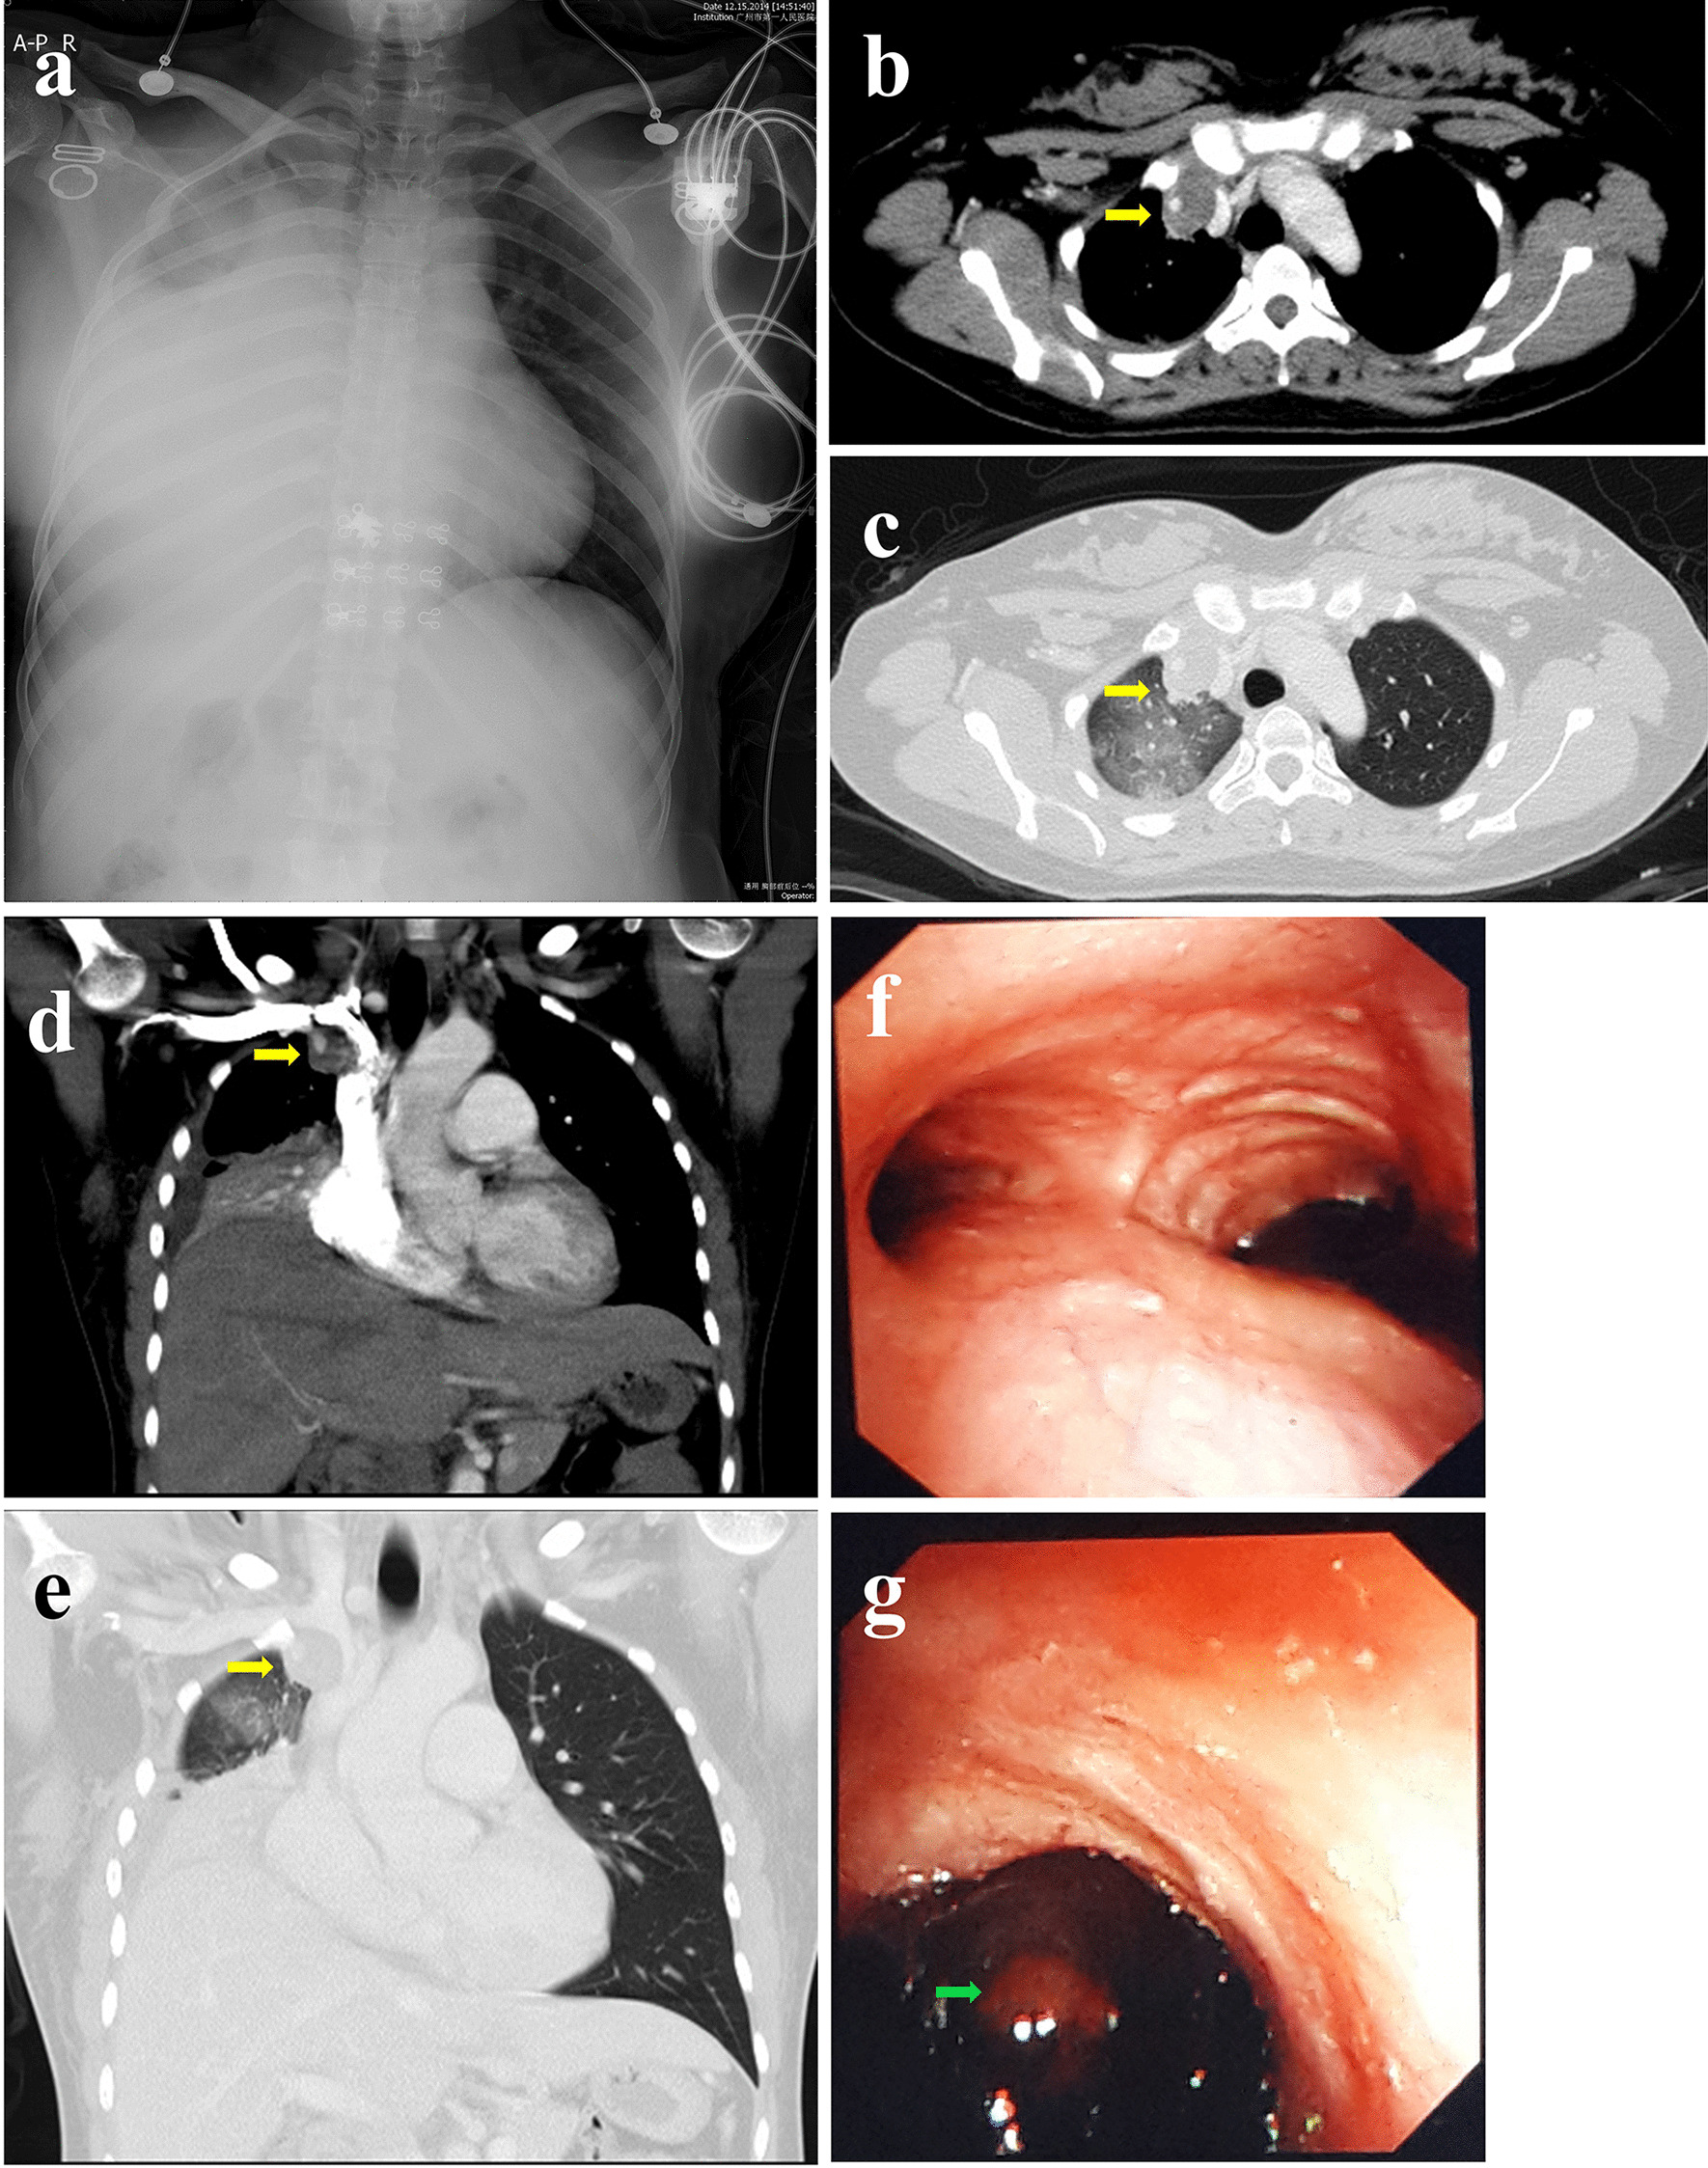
Chest radiograph indicating atelectasis of the right middle and lower lung (a). Computed tomography shows a 2.1 × 3.2 cm^2^ inhomogeneous tumorous lesion (yellow arrow) in the right superior mediastinum on axial views (b mediastinal windows; c lung windows) and coronal views (d mediastinal windows; e lung windows). Bronchoscopy shows tracheal carina (f) and right-side mainstem bronchus (g) blocked by a tumorous protrusion (green arrow) with active hemorrhage
